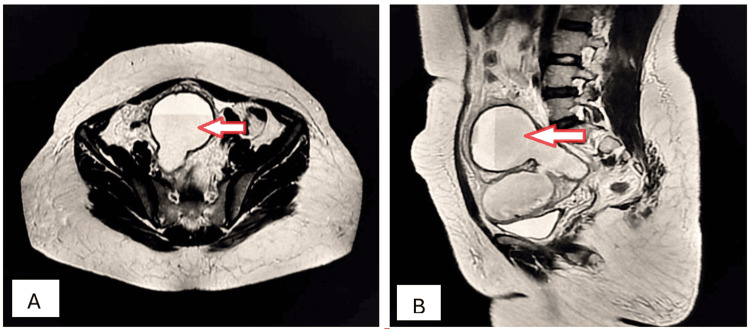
Endometrioma of right ovary (arrows). Axial (A) and sagittal (B) T2W MRI imaging shows a multiloculated, well-circumscribed, cystic mass in the pelvis and a shading sign with a fluid-hem level due to recurrent hemorrhage, consistent with endometrioma. T2W: T2-weighted
